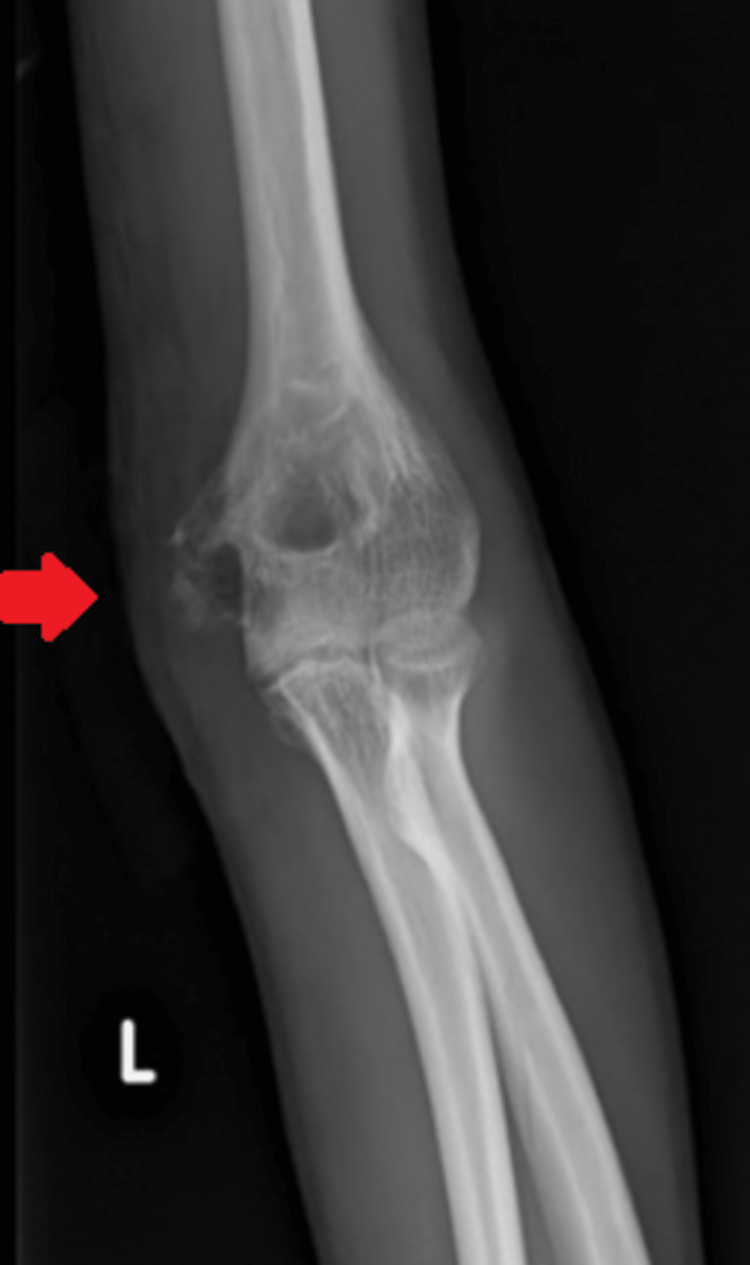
Anteroposterior X-ray of the left elbow joint Arrow showing lytic lesions in the medial epicondyle of the left humerus
